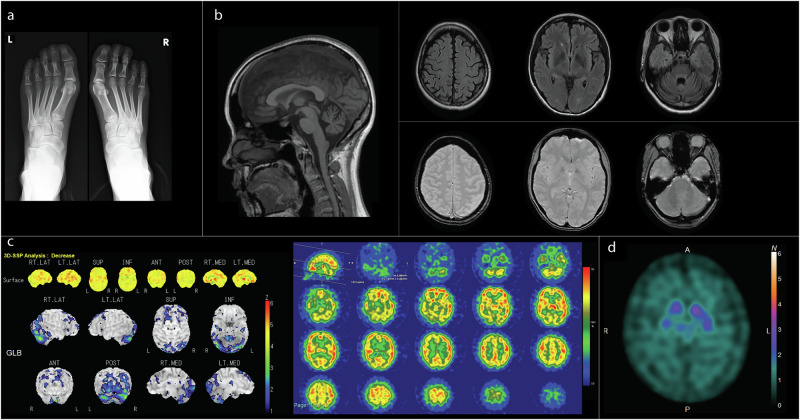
Imaging studies of the patient. a Plain radiographs of both feet. b Magnetic resonance imaging of the brain. Sagittal view of T1-weighted imaging (left) shows cerebellar atrophy. Fluid-attenuated inversion recovery (top right) shows no abnormal high-intensity lesions. T2*-weighted imaging (bottom right) shows no evidence of iron deposition. c Single-photon emission computed tomography with ^123^I-labeled iofetamine. Cerebellar blood flow is decreased. d Dopamine transporter scintigraphy. Decreased uptake, predominantly on the right side, was observed. The uptake was bean-shaped, not the dot-shaped uptake typically observed in Parkinson’s disease
